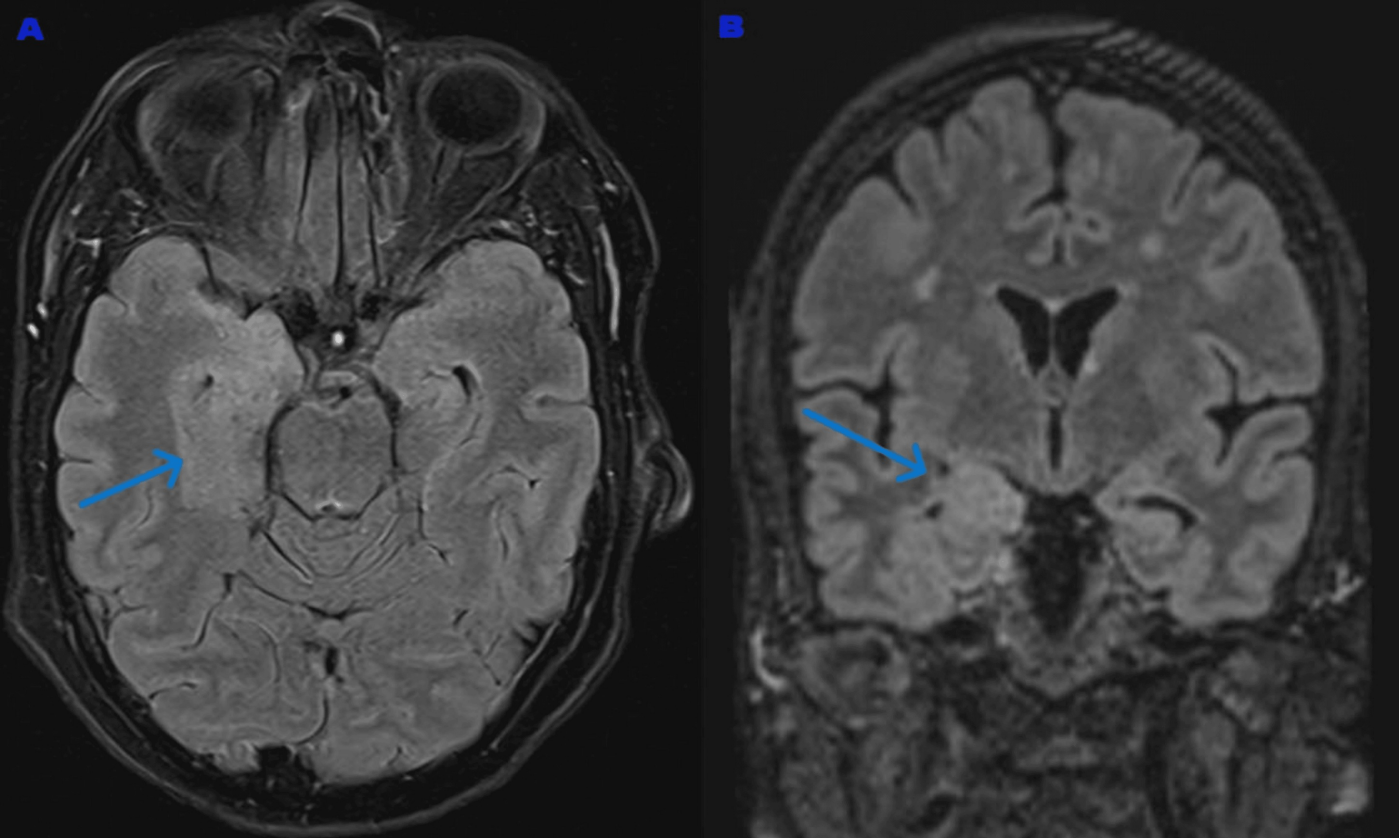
(A) Axial FLAIR MRI of the brain demonstrating right medial temporal lobe hyperintensity (blue arrow). (B) Coronal FLAIR MRI of the brain demonstrating right medial temporal lobe hyperintensity with edema and loss of fissure (blue arrow). MRI: magnetic resonance imaging; FLAIR: fluid-attenuated inversion recovery
